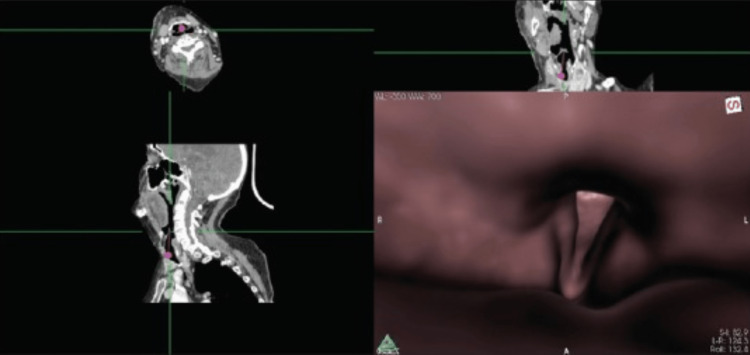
Virtual endoscopy reconstruction of glottis (bottom right) from Computed Tomography images (top and left) Source: Ahmad I, Keane O, Muldoon S. Enhancing airway assessment of patients with head and neck pathology using virtual endoscopy. Indian J Anaesth. 2017 Oct;61(10):782-786. doi: 10.4103/ija.IJA_588_17. PMID: 29242648; PMCID: PMC5664881.
